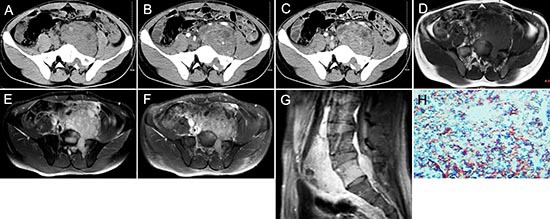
pPNET in a 21-year-old man Precontrast CT images showed a lobular iso-dense mass with necrosis in the left retroperitoneum (A). Enhanced CT images showed the mass had heterogeneous contrast uptake (B-C). Precontrast MRI showed the mass had an ill-defined border that was iso-intense on T1WI (D) and hyper-intense on T2WI (E). Contrast MRI showed the mass had significant and heterogeneous enhancement (F). Sagittal enhanced MRI images showed the mass invaded the 5th lumbar vertebrae and spinal canal (G). The small round tumor cells were positive for CD-99 (H × 100).
